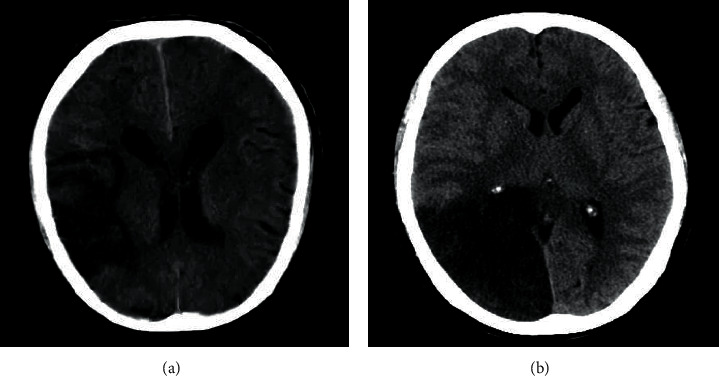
CT images of cerebral infarction.
